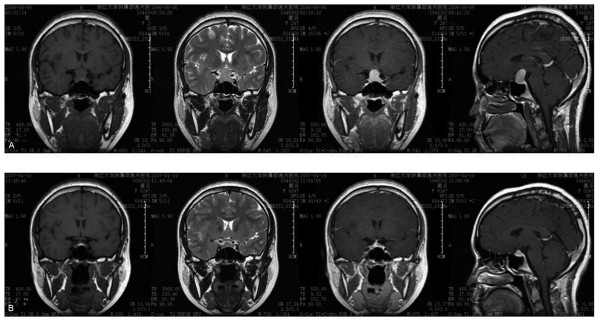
Head MRI before (A) and after thyroxine treatment (B).
